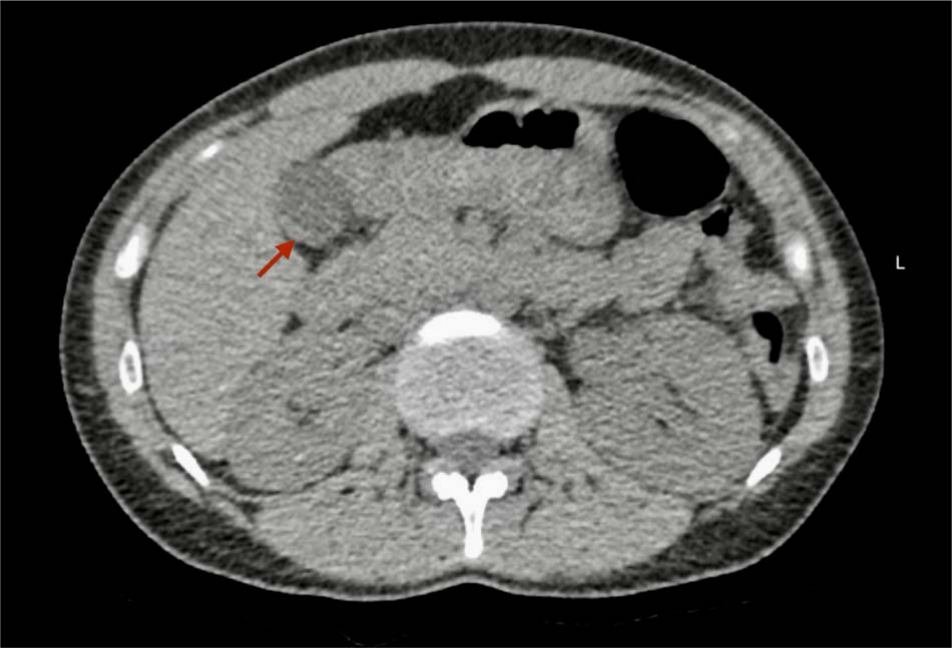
Non-contrast CT scan of the abdomen. The axial plane shows focal thickening of the gallbladder wall (arrow).
